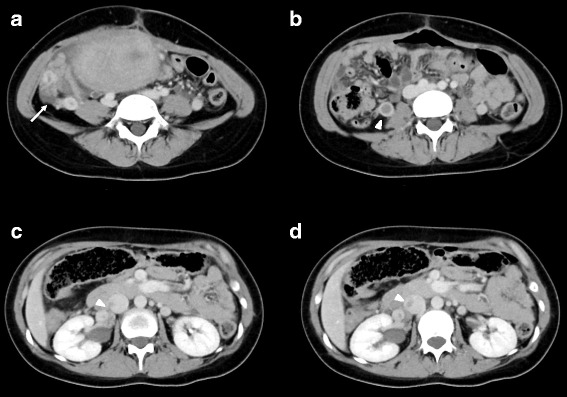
Computed tomography of the right ovarian vein and the inferior vena cava. The right ovary (white arrow) (a). A dilated right ovarian vein (18 × 19 mm) with a hypo-dense filling defect (white arrow head) (b). The right ovarian vein draining into the inferior vena cava (white arrow head) (c). The inferior vena cava with a hypo-dense filling defect indicating thrombi (white arrow head) (d)
